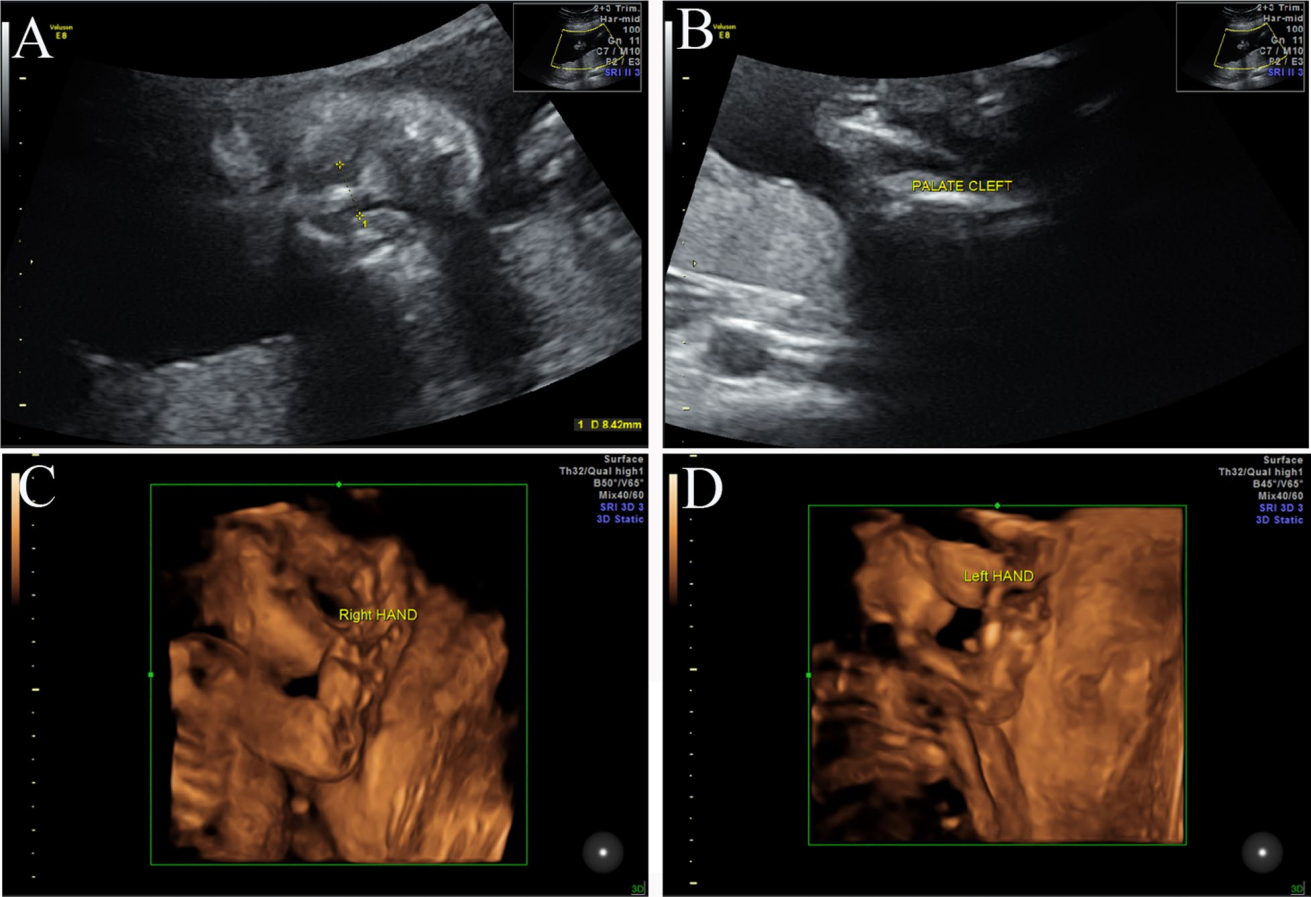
Ultrasound examinations reveal a bilateral cleft lip and cleft palate in the fetus (A, B), the absence of the right thumb and the presence of an appendicular thumb on the left (C, D)
